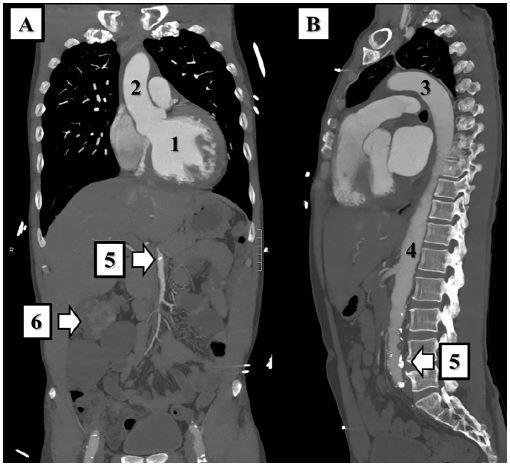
Coronal (A) and sagittal (B) views of the patient’s computed tomography angiogram of the chest, abdomen, and pelvis. The patient’s cardiomegaly can be seen (1), but there is no evidence of dissection at the aortic root (2), arch (3), or descending aorta (4). Calcifications within the abdominal vasculature (5) suggest atherosclerosis. Evidence of the patient’s known polycystic kidney disease was seen (6).
